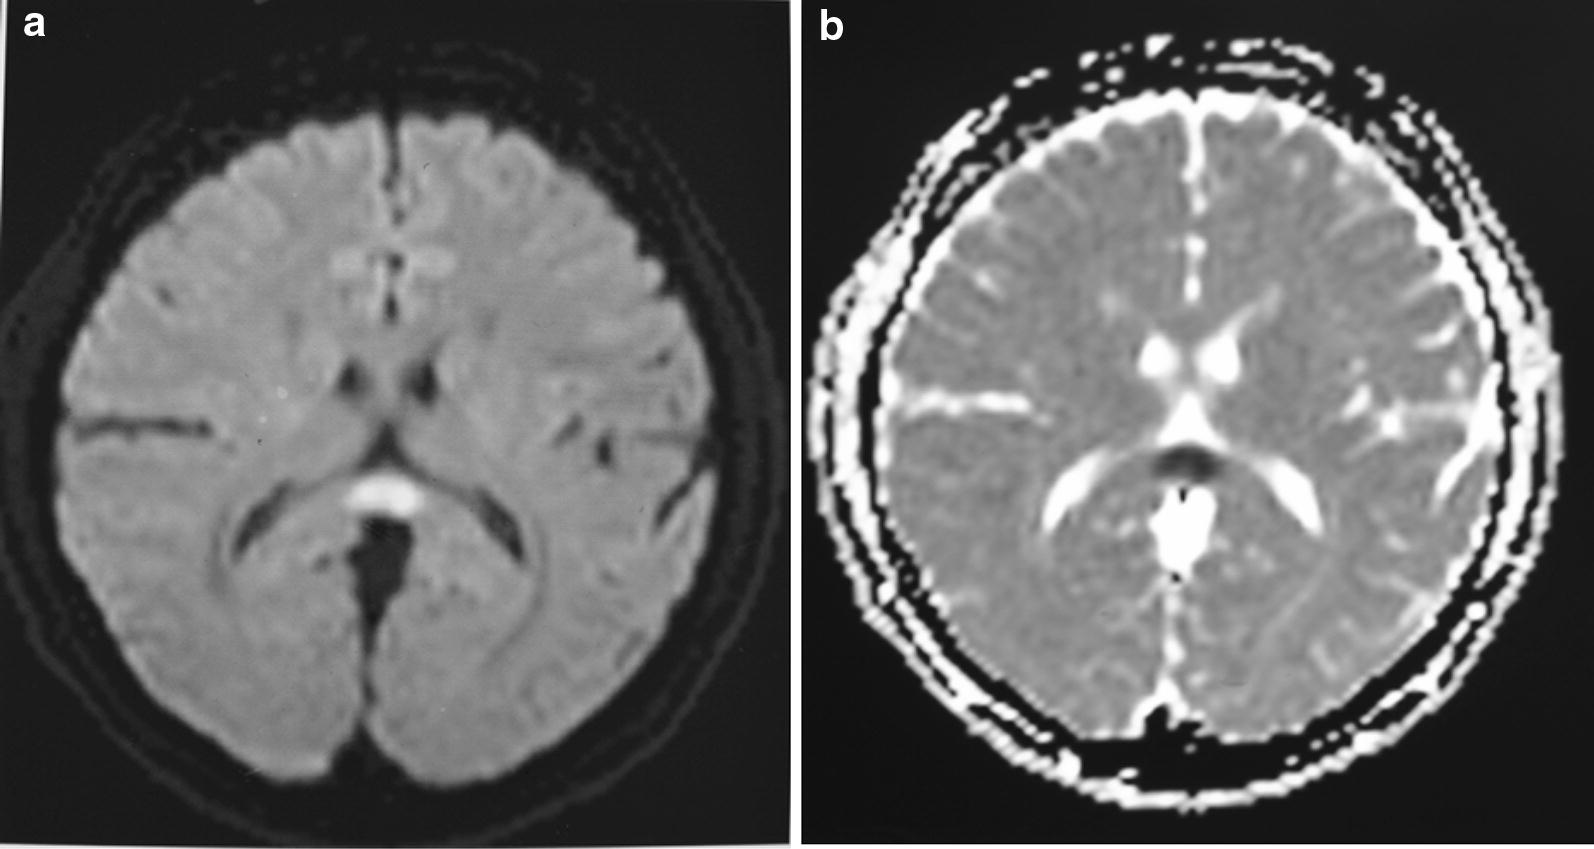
Initial MRI of brain (axial view) flair (a) and diffusion-weighted imaging (b) showing a lesion in the central splenium of corpus callosum
